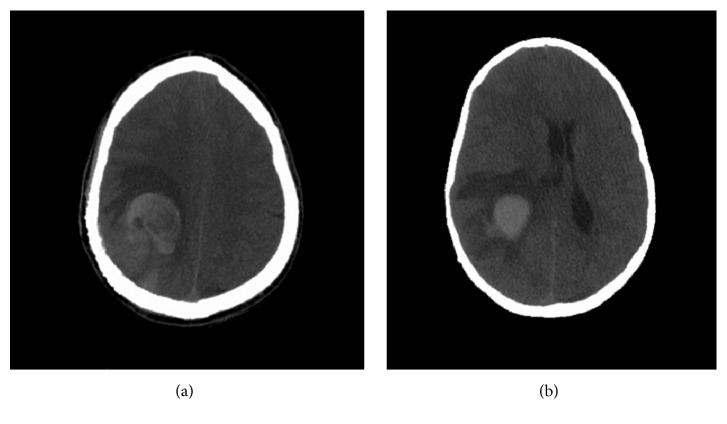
Axial CT of head for patient demonstrates right parietal heterogeneous, hyperdense mass with a large medial hematoma.
